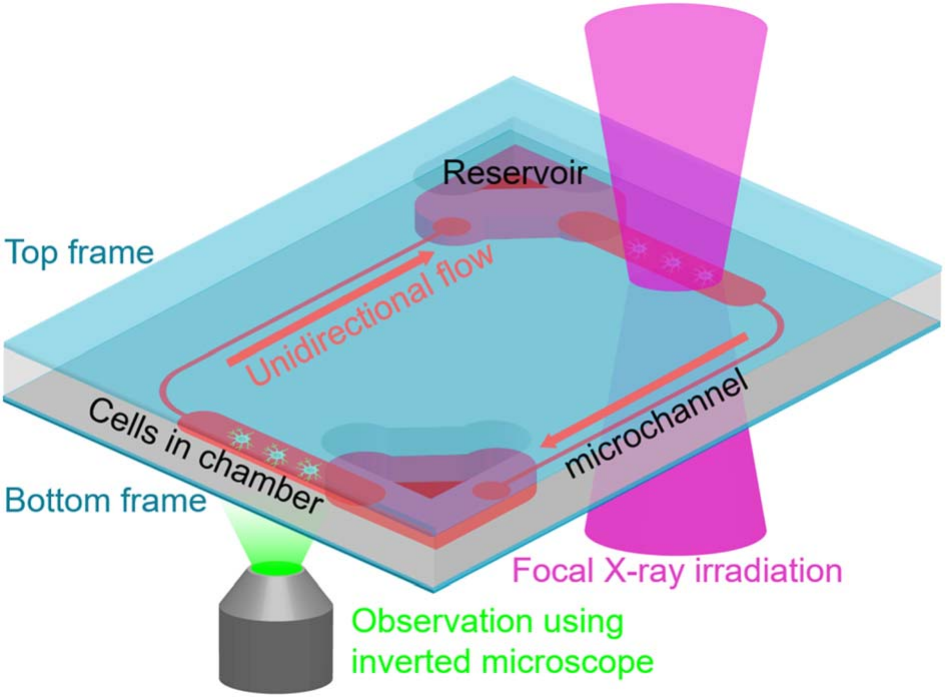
Closed, pumpless microphysiological system (CPMS). The CPMS was designed for isolating the space for cultured cells and circulating culture medium from the outside by sealing it with top and bottom thin glass frames. The culture medium was circulated unidirectionally by a gravity-driven passive flow using the water head pressure generated by tilting the proposed system. Since no driving mechanisms, such as pumps or active valves, are used in the isolated space, the excess space is minimized. In this system, cells were cultured on a bottom-thin glass frame. It allowed observation of the cells and focal irradiation of X-rays without exposing the culture medium to the outside.
